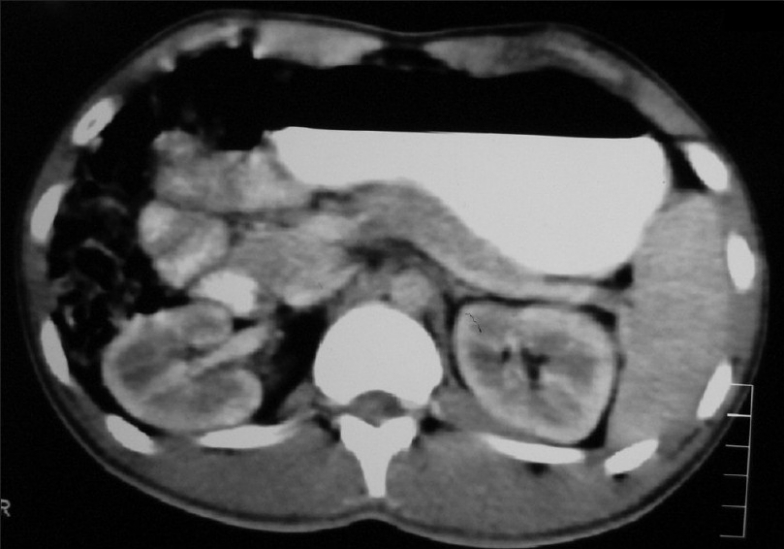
CT abdomen (2008) shows reduction of para aortic lymphnodes to sub centimeter size after chemo
